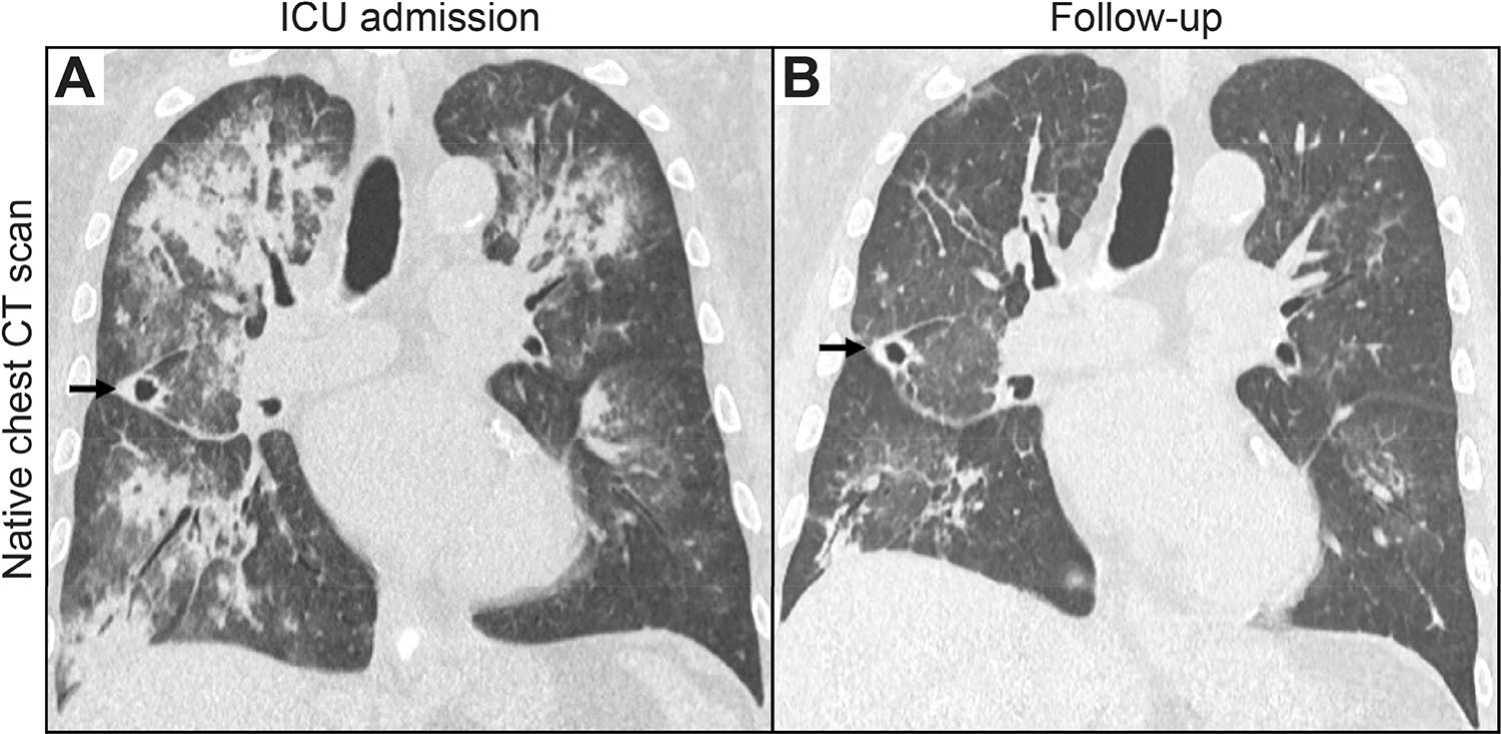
Native CT scans of the chest at the time of ICU admission and during follow-up. A At the time of admission, a CT scan confirmed diffuse pulmonary hemorrhage in the upper lobes and right lower lobe with widespread areas of consolidation with surrounding ground glass opacities and crazy-paving as well as a cavitating nodule (arrow) in the middle lobe consistent with GPA. B A follow-up CT scan 2 weeks after ICU admission revealed regression of the diffuse pulmonary hemorrhage with residual linear areas of consolidation and focal ground glass opacities. The cavitating nodule in the middle lobe remained unchanged (arrow). *CT* computed tomography, *GPA* granulomatosis with polyangiitis, *ICU* intensive care unit
